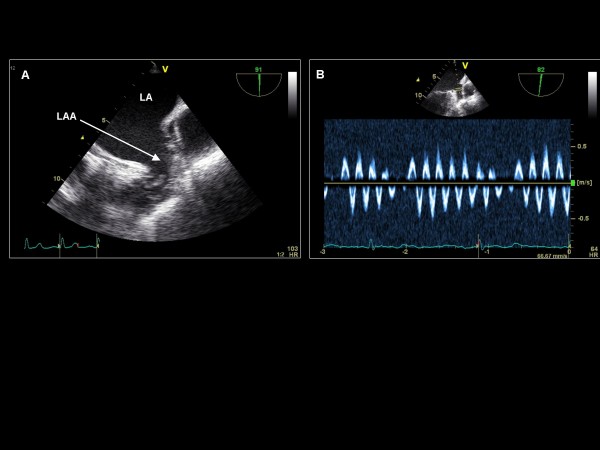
Imaging plane 90°, (A) The LAA is identified. (B) Pulse wave Doppler applied to the LAA demonstrating emptying velocities in a patient with atrial flutter.
